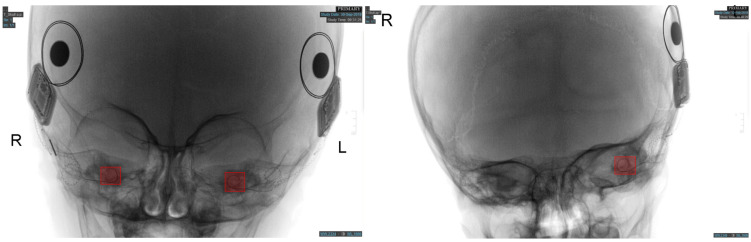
Representative early postoperative CI radiographs (standardized inverted view) with manually annotated ROI bounding boxes around the electrode array: bilateral (left) and unilateral (right) implantations. R and L denote right and left ears, respectively. Red boxes indicate the manually drawn regions of interest (ROI) encompassing the electrode array for each implant.
